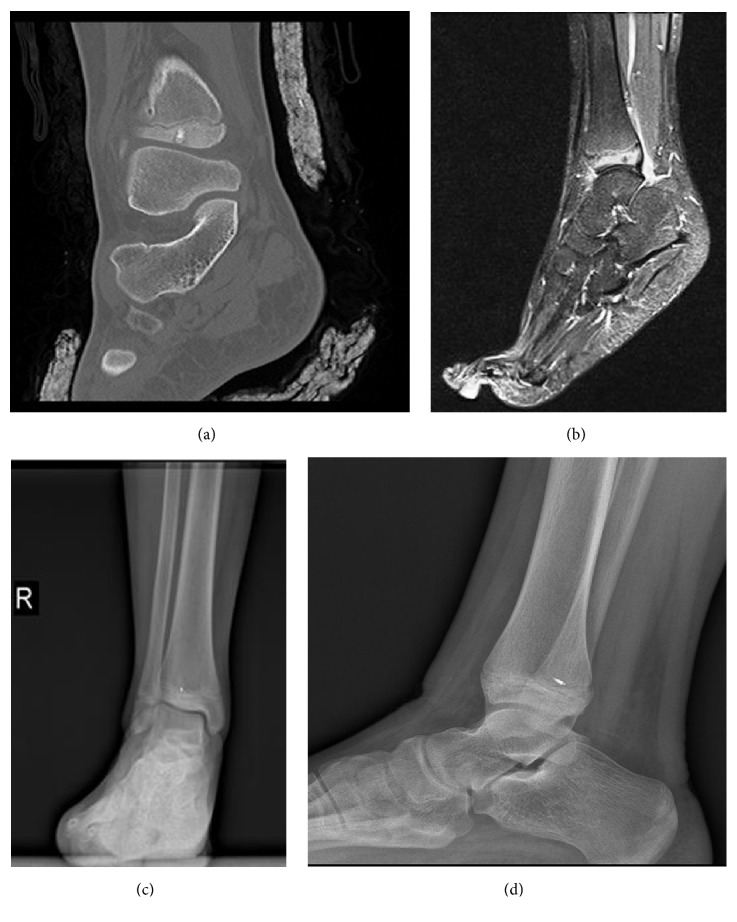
(a) and (b): CT and T2 MRI sections of the lesion located in distal tibial physis. (c) and (d): direct graph images of the probe tip broken after the procedure.
